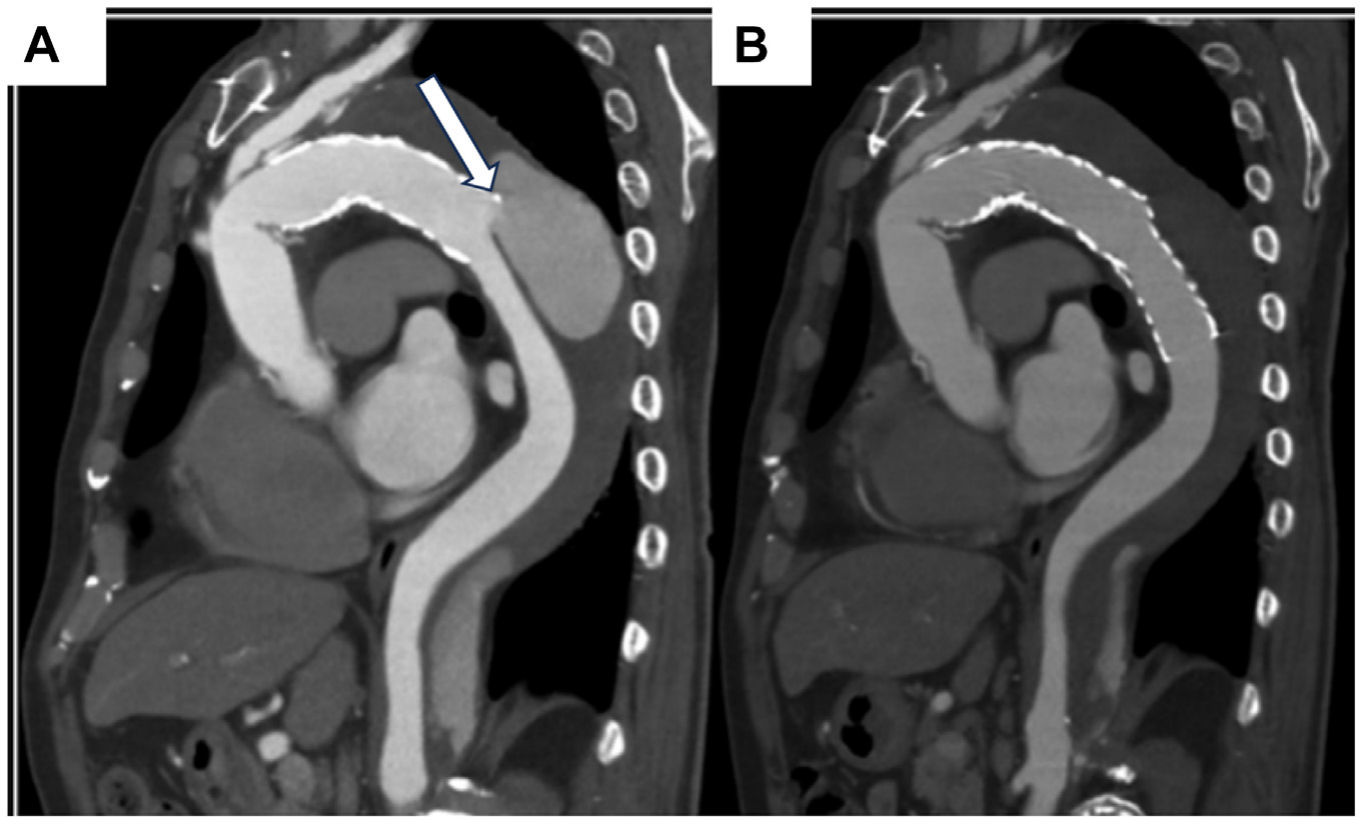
(A) Arrow pointing at distal stent graft-induced new entry (SINE) after thoracic endovascular aortic repair (TEVAR) (B) Follow-up computed tomography angiography (CTA) after TEVAR distal extension covering the SINE. (Reproduced from Nomura Y, Tonoki S, Kawashima M, et al. Distal stent graft-induced new entry after total arch replacement with frozen elephant trunk for aortic dissection. Ann Vasc Dis 2021;14:362-367.).
